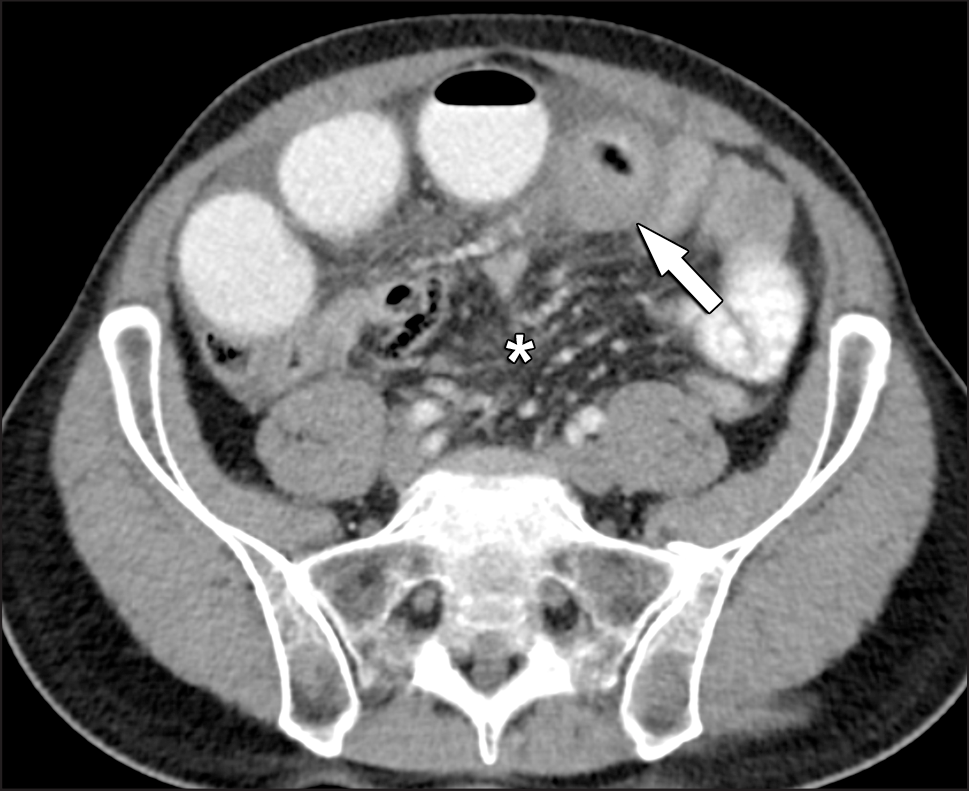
Axial view of contrast-enhanced CT reveals circumferential small bowel wall distention (arrow) and engorged mesenteric vessels (*).
